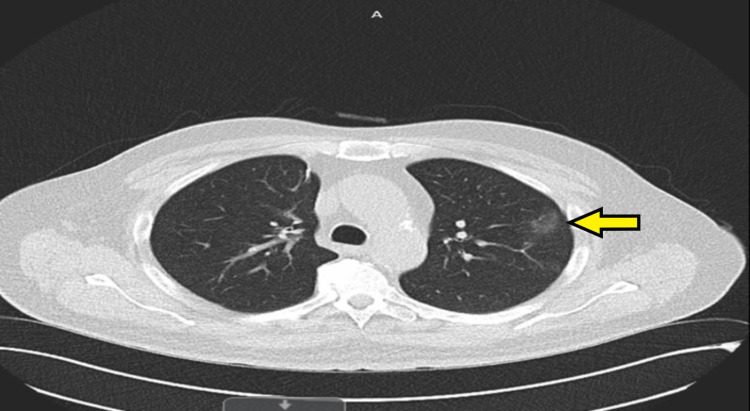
Axial CT scan of the chest demonstrating significant resolution of the left upper lobe nodule, with only a dense linear band of tissue remaining (yellow arrow) CT: computed tomography
